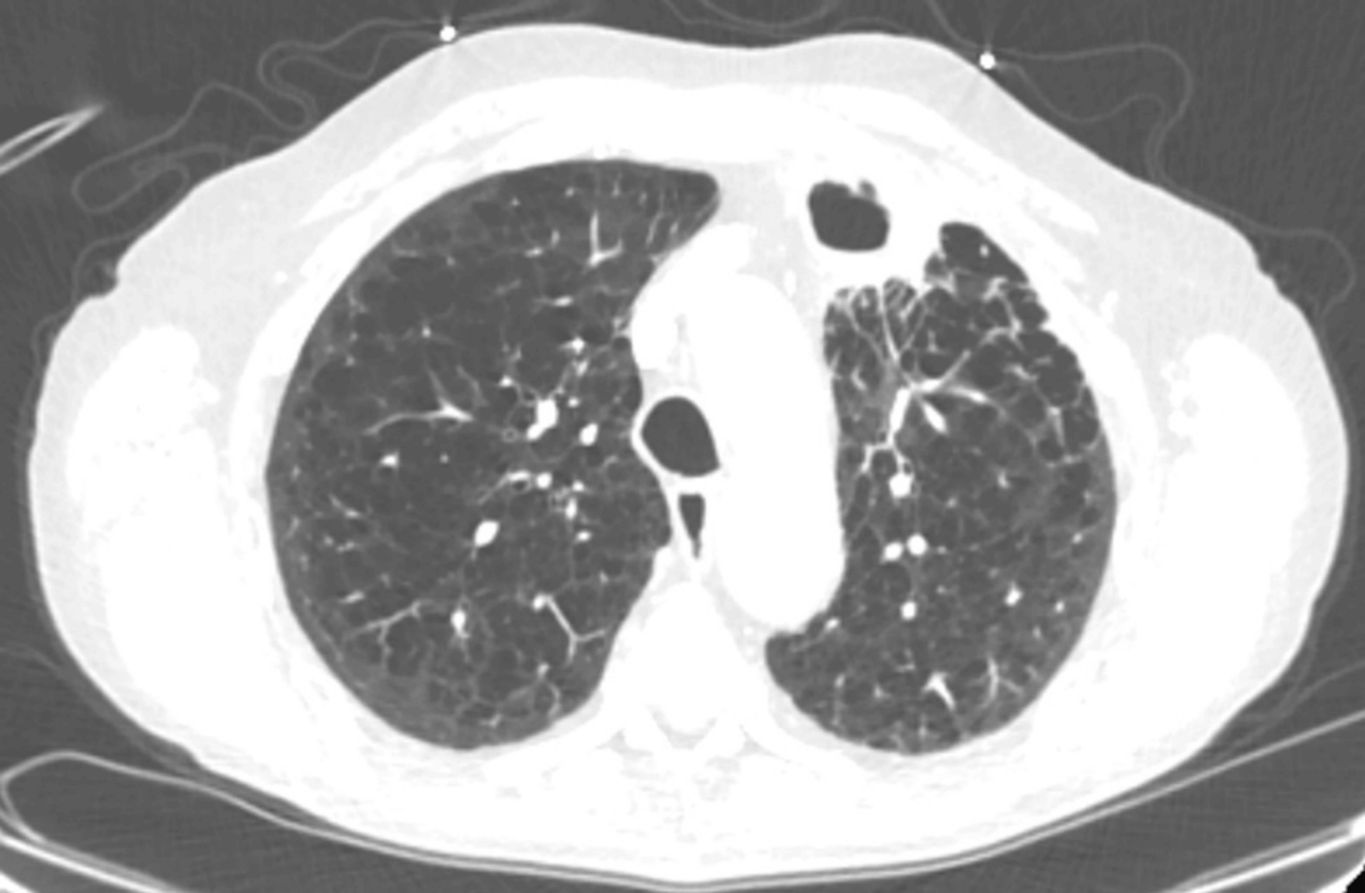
A contrast-enhanced CT scan of the chest The scan showing a cavitary lesion in the anterior segment of the left upper lobe measuring 3.8 cm x 4 cm in the antero-posterior by transverse dimensions, respectively.
